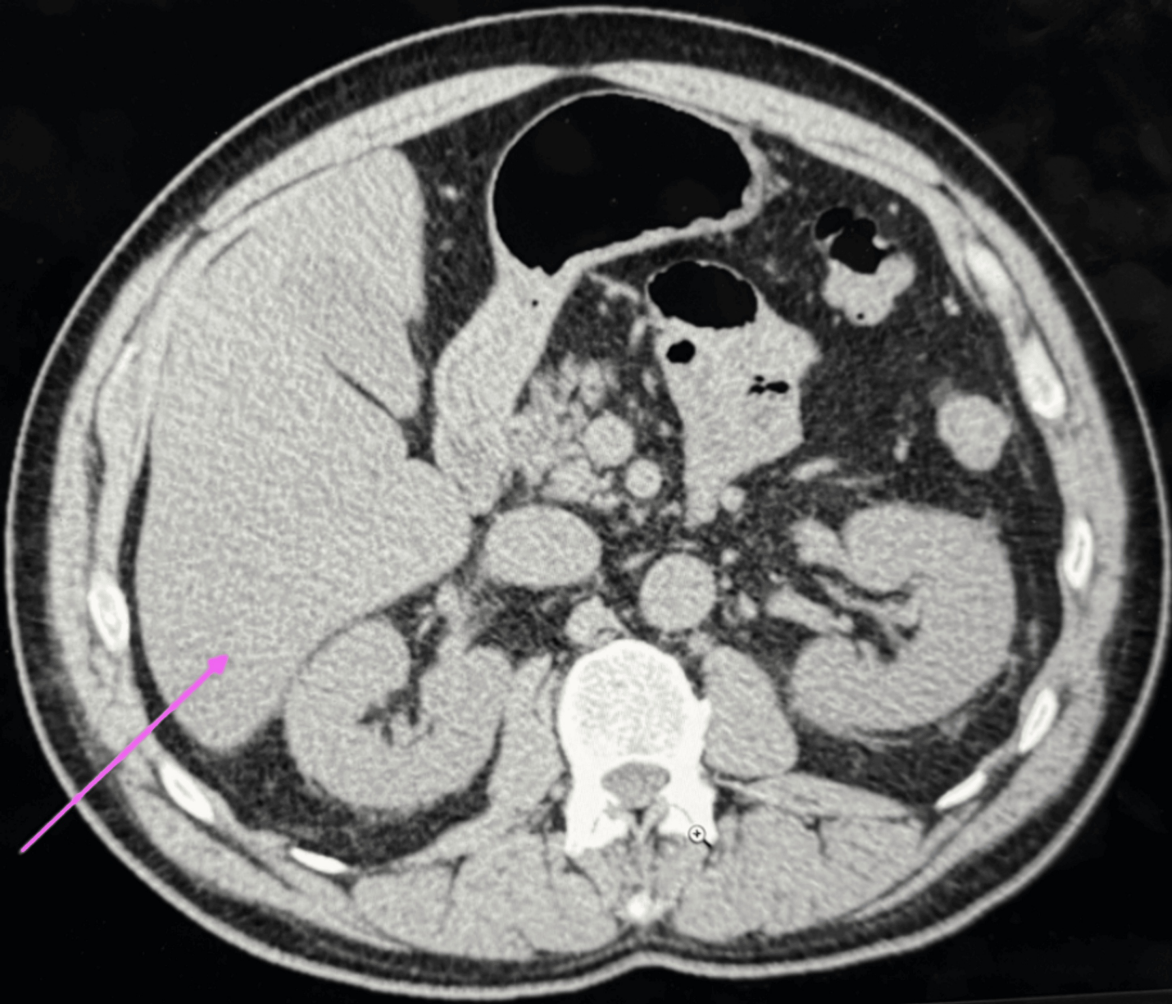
Computed tomography of thorax, abdomen, and pelvis for patient showing no biliary obstruction Pink arrow indicates that the liver appears normal.
